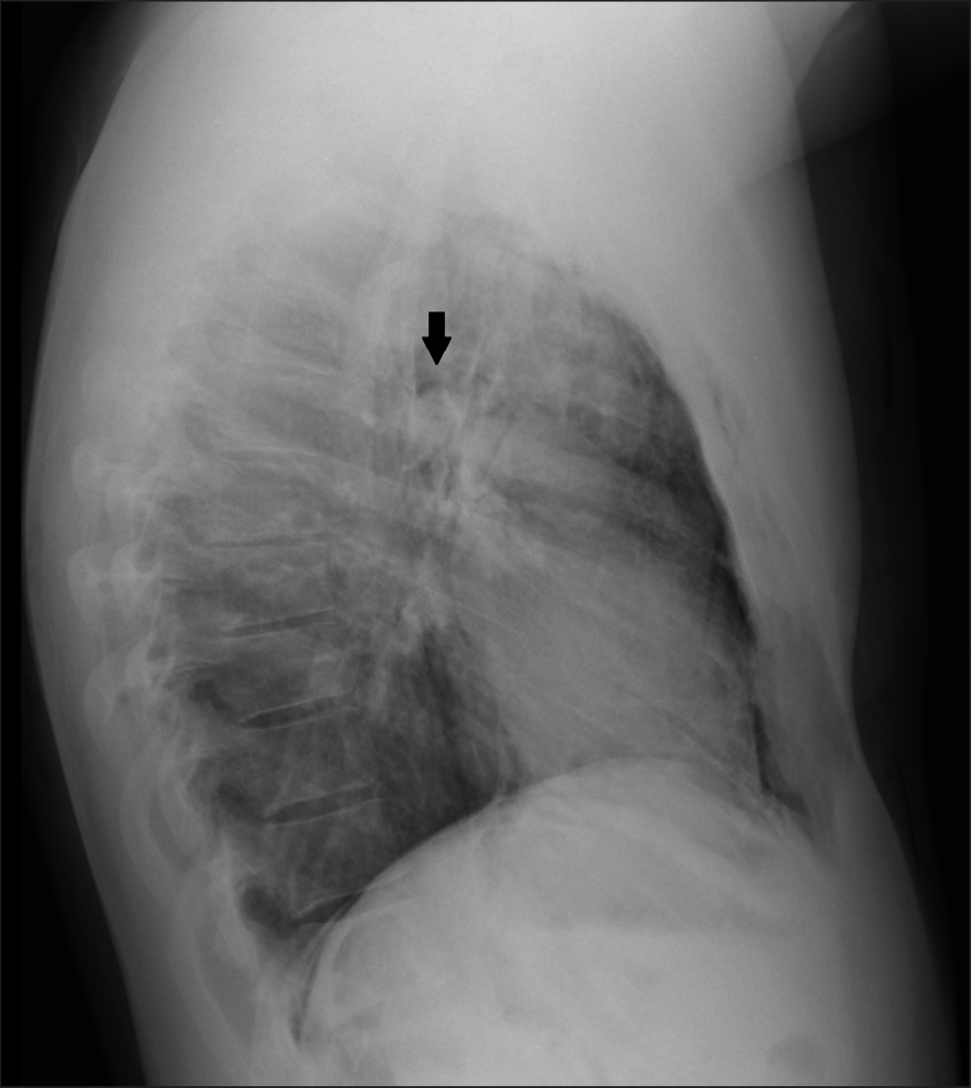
Lateral chest radiograph showing a well-defined lucency surrounding the right pulmonary artery, also known as the ring around the artery sign (solid black arrow).
